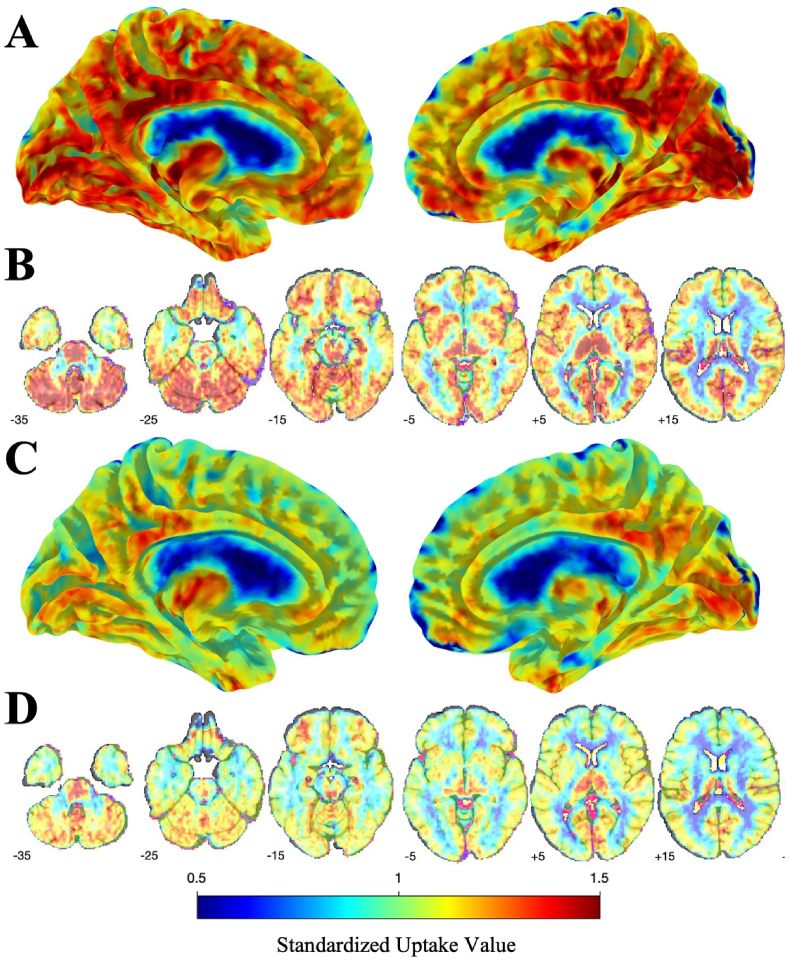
Fused [^18^F]-FEPPA and Structural Image showing differences by standardized uptake values (SUV) in participants with persistent Brain Fog following COVID-19 infection as compared to pre-pandemic controls. Panel A shows TSPO SUV in N-PASC + participants, mapped to the cerebral surface. Panel B shows [^18^F]-FEPPA SUV values mapped to the cerebral parenchyma. Panel C shows [^18^F]-FEPPA SUV values for pre-pandemic controls mapped onto the cerebral surface. Panel D shows similar values for [^18^F]-FEPPA SUV values among pre-pandemic controls throughout the cerebral parenchyma.
